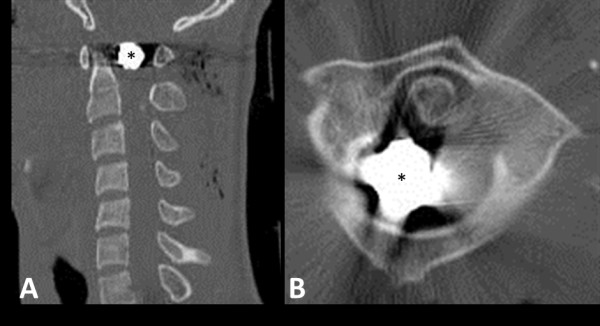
Radiographic imaging of a retained bullet fragment in the high-cervical spine. Sagittal (A) and axial (B) computed tomography (CT) images show the relationship of the fragment (designated by *) to the arch of C1 and the dens of C2. Note the fracture of the right posterior arch of C1 (arrow).
